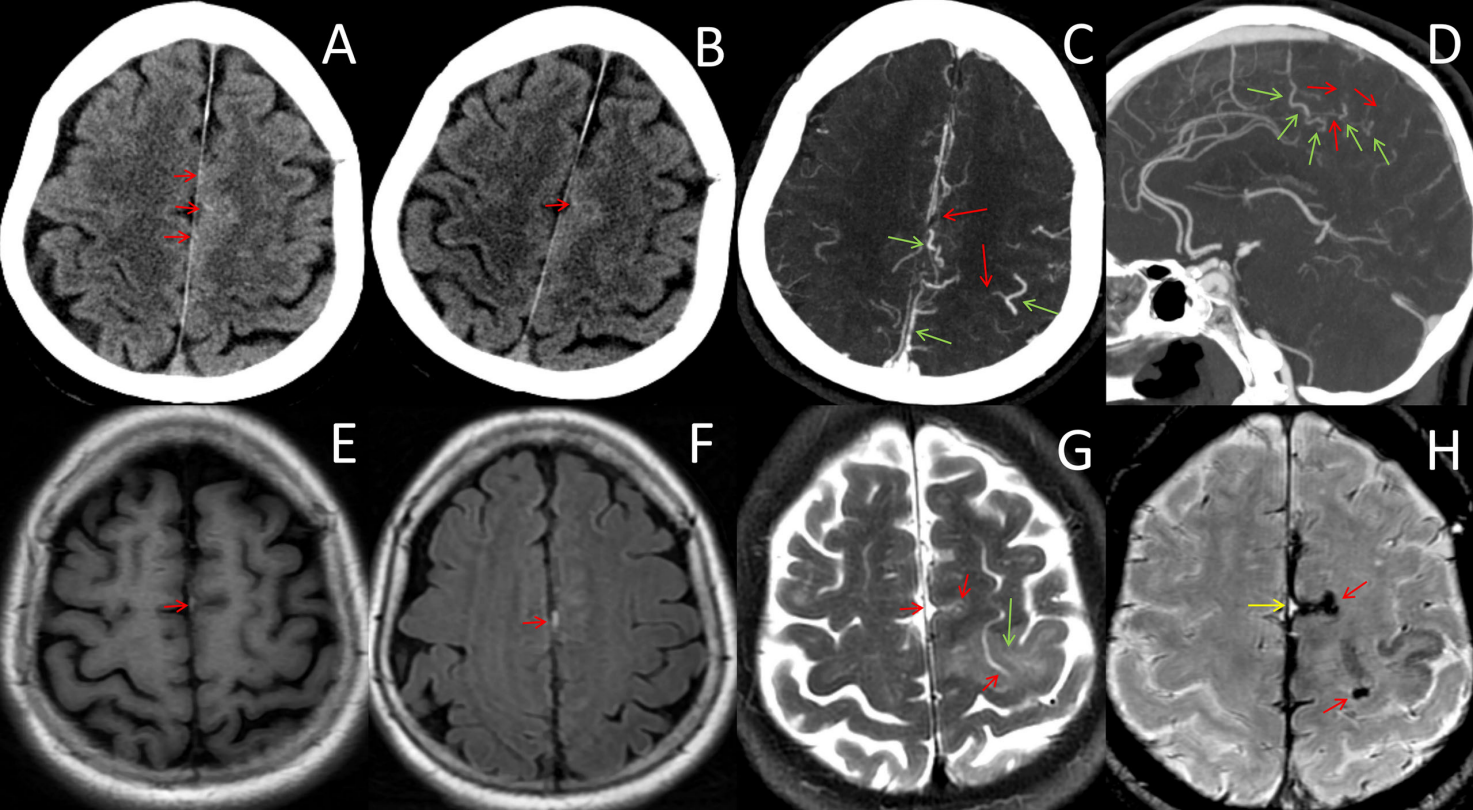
Neuroradiological data from onset to day 4. (A, B) CT images before and after intravenous thrombolysis showed no abnormalities except punctate hyperdensities within the left frontal sulci (red arrows). (C, D) CT angiography on day 4 showed filling defects of cortical veins (red arrows) and compensatory expansion of the adjacent veins (green arrows). (E, F) MRI on day 3 showed hyperintense T1WI and FLAIR lesions neighboring the falx cerebri and the left parietal cortex (red arrows), which were initially diagnosed as subarachnoid hemorrhages, indicating subacute thrombosis in the cortical veins. (G) MRI on day 4 showed venous infarction in the frontal lobe (green arrow) and the absence of the flowing-void effect in the cortical veins (red arrows). (H) Susceptibility-weighted imaging on day 4 showed curvilinear, serpentine, and exaggerated susceptibility within the sulcus, which was suggestive of thrombosed veins during the acute (red arrows) and subacute phases (greed arrow).
